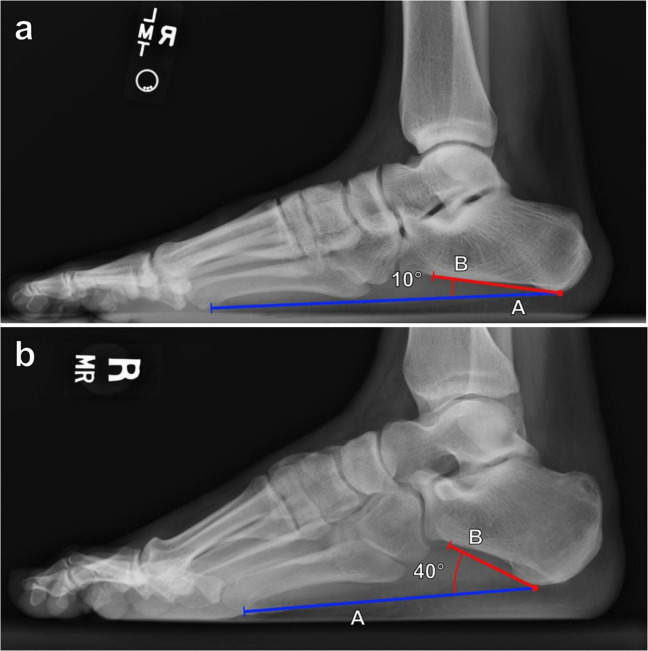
Measurement of the calcaneal inclination (pitch) angle on lateral weightbearing radiograph of the foot. The angle is formed between a horizontal line (A, blue line) from the calcaneal tuberosity to the plantar surface of the 5th metatarsal head, and a line (B, red line) along the inferior surface of the calcaneus. Reported normal values are variable although 20°–30° is often considered normal. A calcaneal inclination angle measuring < 20° is consistent with pes planus, while an angle > 30° suggests of pes cavus. a Pes planus in a 24-year-old male patient with a 10° calcaneal inclination angle. b Pes cavus in a 30-year-old female patient with a 40° calcaneal inclination angle
